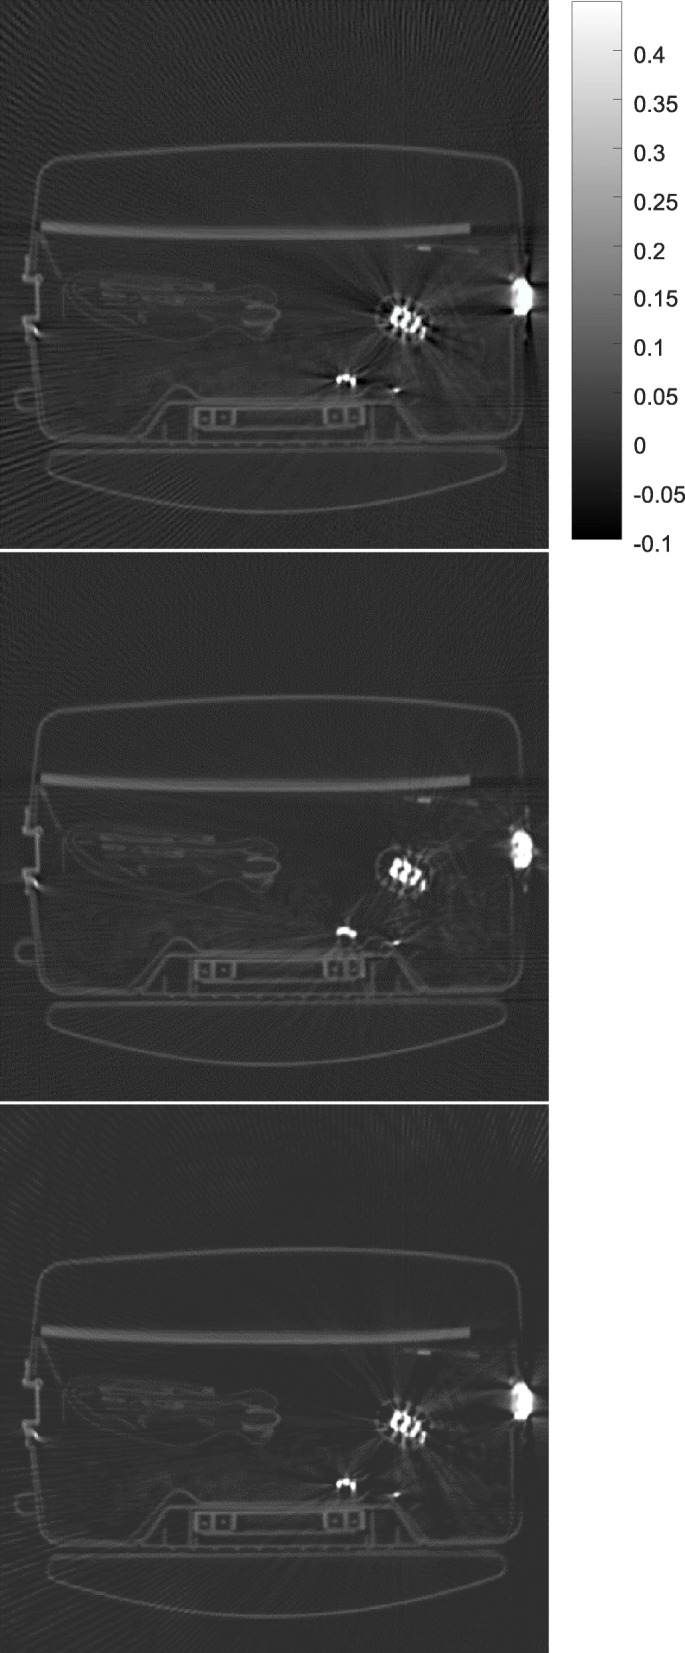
CT image reconstruction of airport bag #5. Top: the raw FBP reconstruction; Middle: the reconstruction using the proposed algorithm; Bottom: the raw FBP reconstruction with all negative pixel values replaced by zeros
